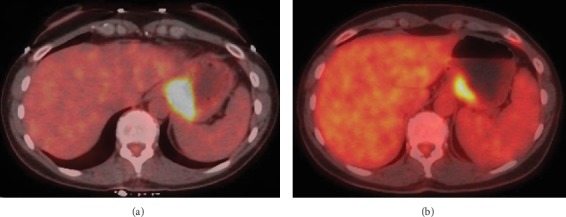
Initial axial fused PET/CT image (a) showing robust hypermetabolism of the gastric cardia (max SUV 10.4) with subsequent axial fused PET/CT image (b.) following neoadjuvant therapy with reduced size and uptake (SUV 4.3), consistent with 60% reduction in FDG concentration.
